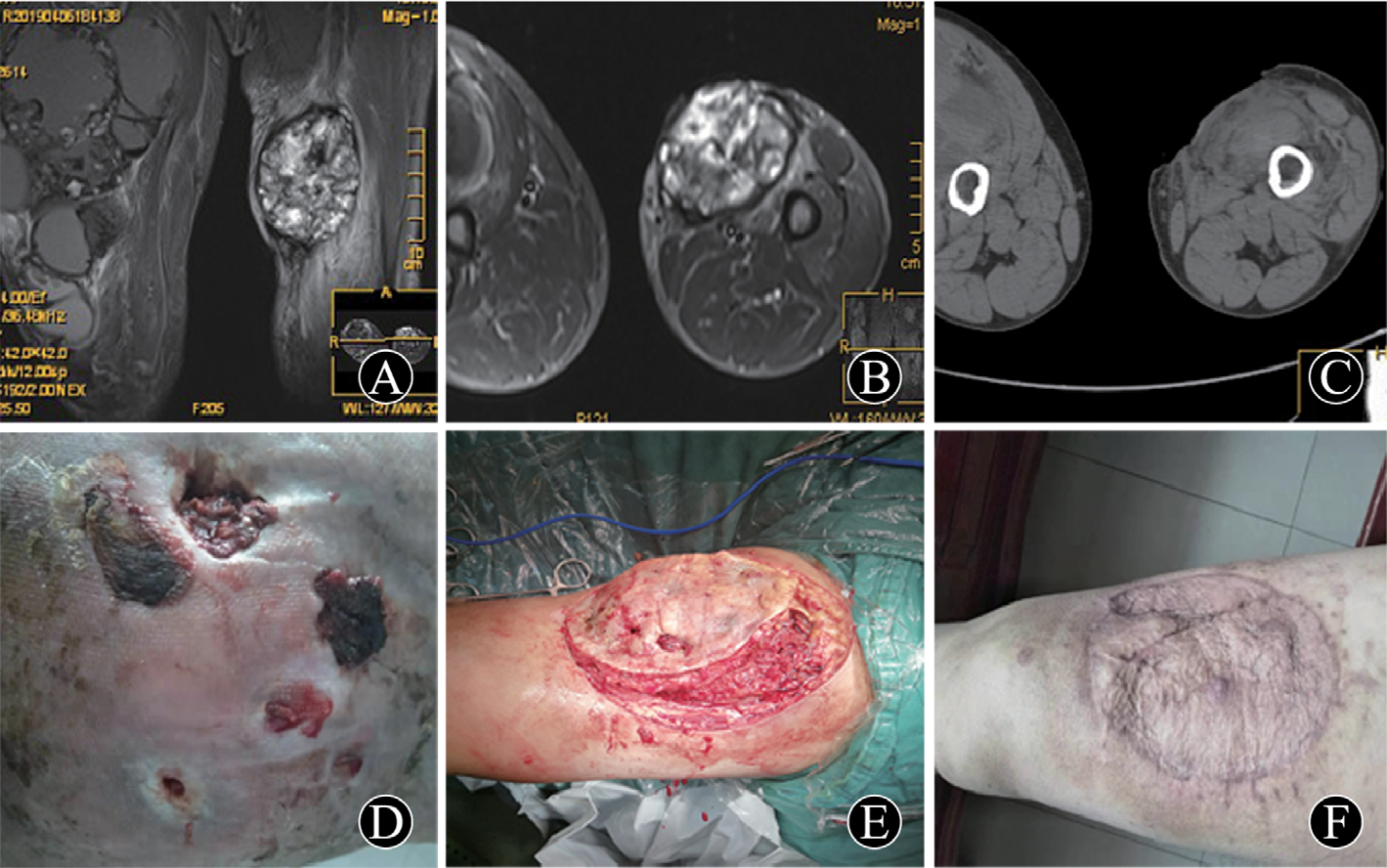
A 51‐year‐old man (patient 6), who had hemophilic pseudotumor in both thighs for 6 years, skin ulceration and fistula formation in the left thigh for 2 years. (A) Preoperative MRI T2 image in the coronal plane. (B) Preoperative MRI T2 image in the transverse plane, which showed heterogeneous signal intensity. (C) Preoperative CT image showed skin and soft tissue defect, but no bone destruction. (D) Preoperative skin ulceration and fistula formation in the left thigh. (E) Intraoperative photograph. (F) The wound healed and postoperative photograph taken at 9 months after surgery.
